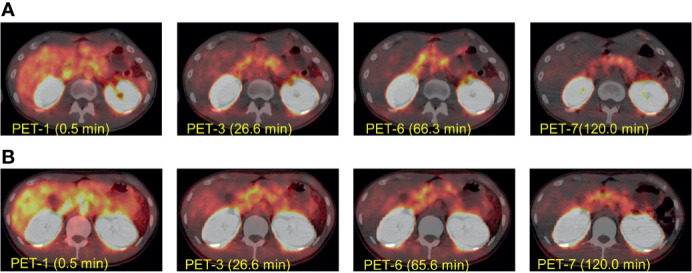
The representative transverse [^18^F]FB(ePEG12)12-exendin-4 (^18^F-Ex4) PET/CT images. The transverse [^18^F]FB(ePEG12)12-exendin-4 (^18^F-Ex4) PET/CT images of Case 2 (A) and Case 3 (B). The first PET scan was performed immediately after ^18^F-Ex4 administration, and following five-time scans consisting of 2 min/frame × 5 frames (PET 1–6) were undertaken. The final scan was started at 2 h after administration of ^18^F-Ex4 (PET 7). The numbers in parentheses indicate the time after ^18^F-Ex4 administration.
